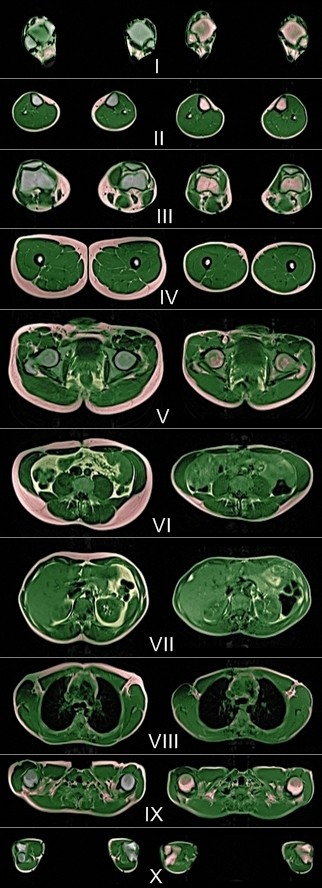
Semiautomatic separation of adipose bone marrow: selected slices from whole body MRI of a 32-year-old male finisher of TEFR09. I: ankles, II: middle of lower legs, III: knees, IV: middle of upper legs, V: hip/pelvis, VI: umbilical level, VII: upper abdomen, VIII: heart/mediastinum, IX: shoulder girth, X: elbows. Left row: before start (t0), green: TLT, red: SAST, yellow: VAT+INF, blue: ABM. Right row: after 4,120 km of running (t5), green: TLT, red: SAT (=SAST + ABM), yellow: VAT + INF. ABM, adipose bone marrow; INF, intraluminal nutrition fat; MRI, magnetic resonance imaging; SAST, somatic adipose soft tissue; SAT, somatic adipose tissue; TEFR09, Transeurope Footrace 2009; TLT, total lean tissue; VAT, visceral adipose tissue.
